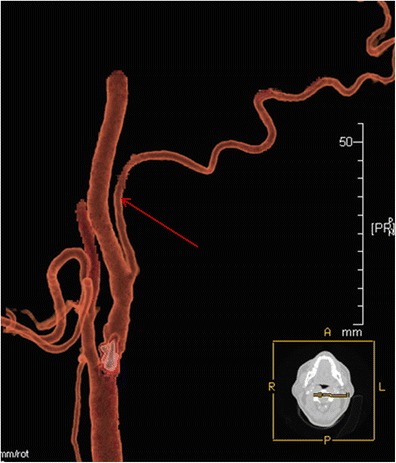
CTA (VRT 3D reconstructions) shows a case of the origin of the occipital artery from the ICA (*red arrow*)
